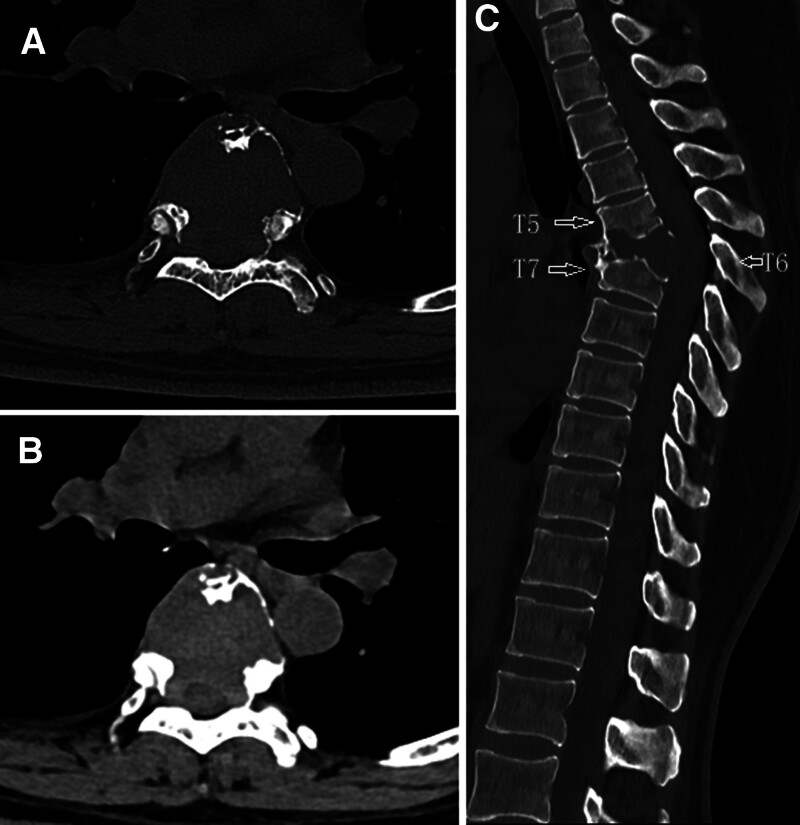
CT axial bone window (A) and soft tissue window (B) showing vertebral body bony destruction. Sagittal reconstruction (C) showing that the T5–7 vertebral bodies and intervertebral discs have been destroyed, causing thoracic kyphosis. CT = computed tomography.
